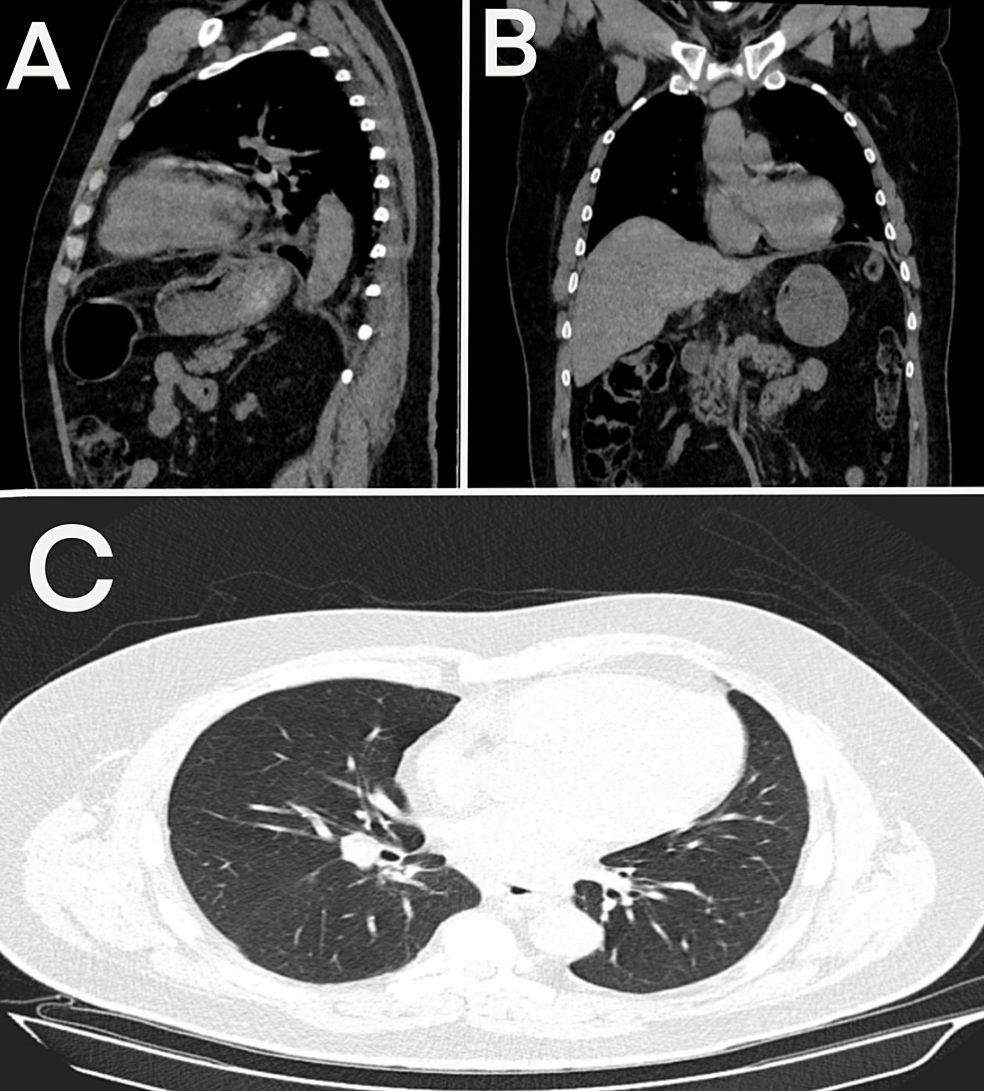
Postoperative computed tomography after hernia repair A: Sagittal view; B: coronal view; C: axial view
